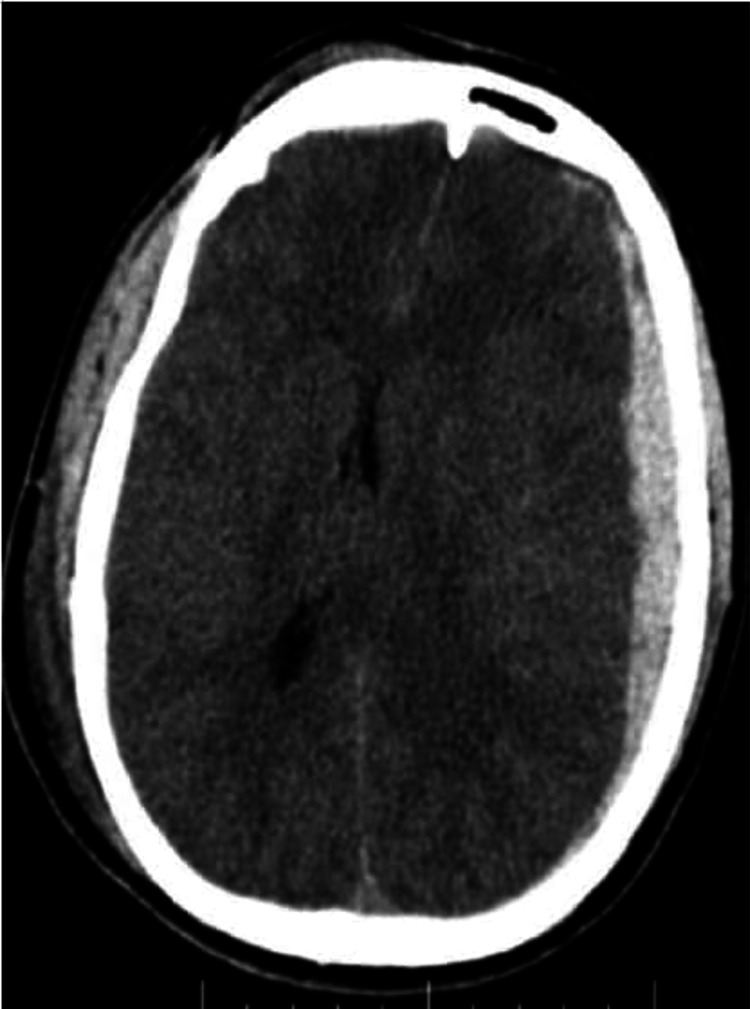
Axial NECT shows subdural hemorrhage in the left cerebral hemisphere, with mass effect compressing basal cisterns and lateral ventricles, midline shift more than 5mm, subarachnoid hemorrhage noted - Rotterdam score 5 NECT: non-contrast-enhanced computed tomography
